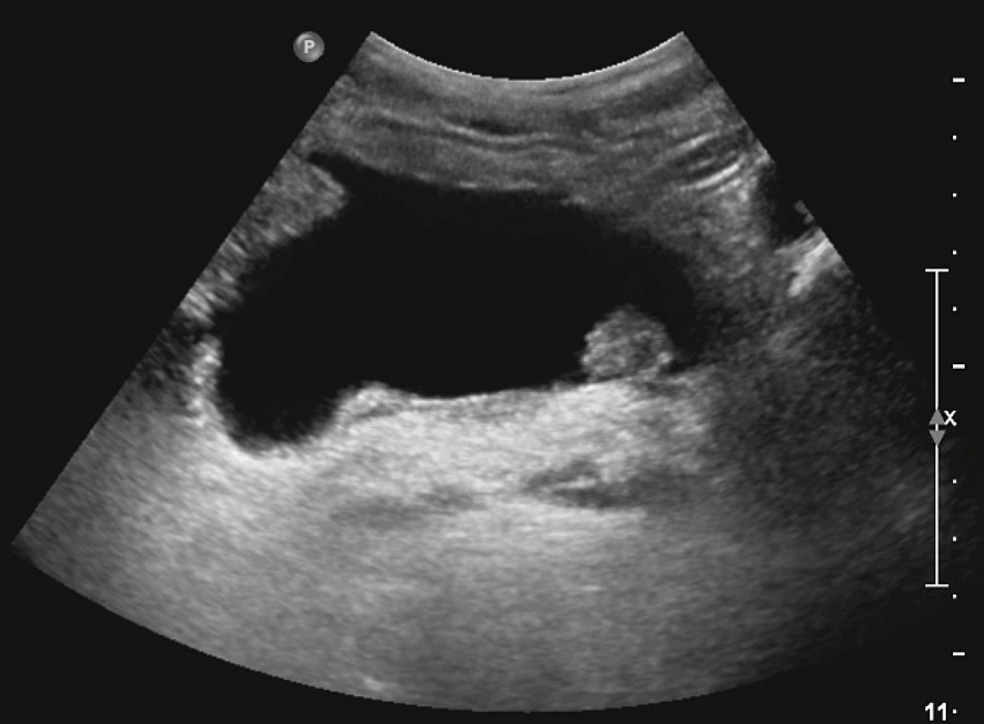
Ultrasound of the bladder showing a lobulated polypoid lesion
